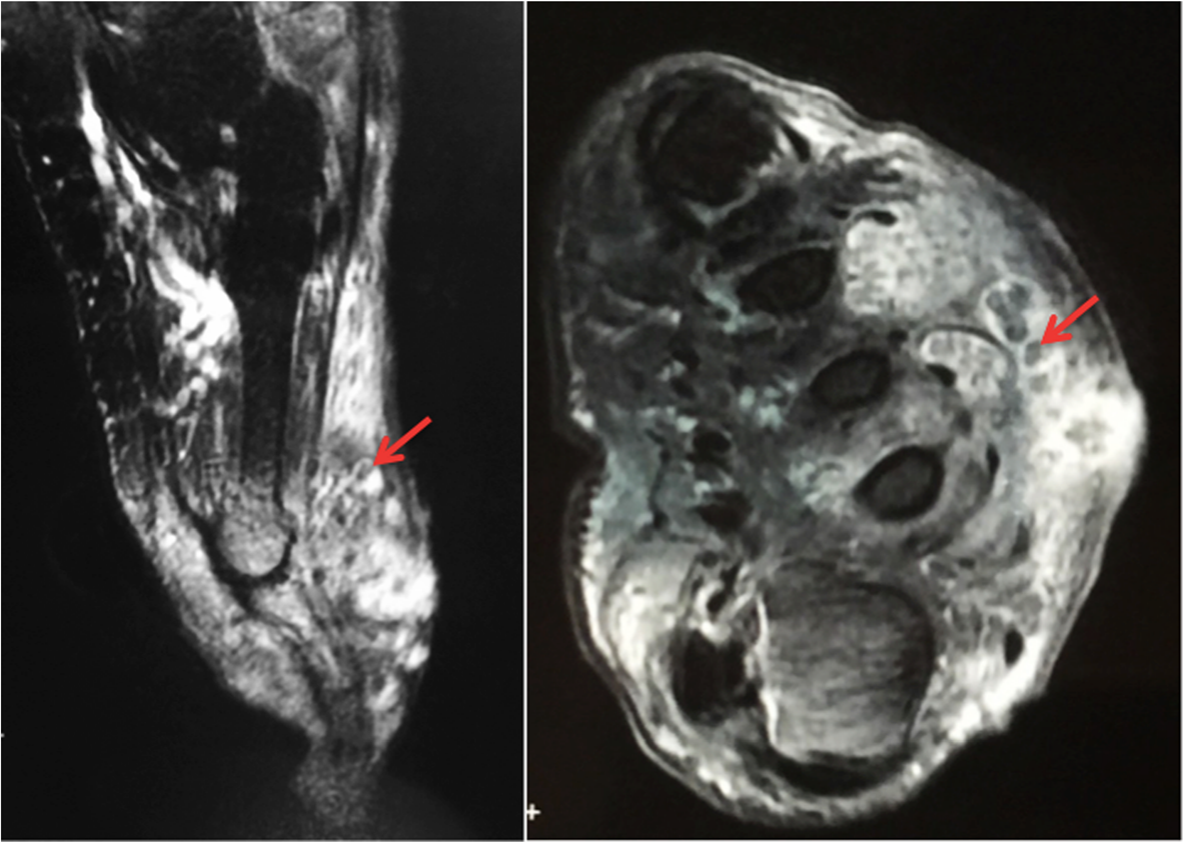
Magnetic resonance imaging scan of the right foot showing the « dot in circle » sign (arrow). a-Sagittal view; b-Transversal view
